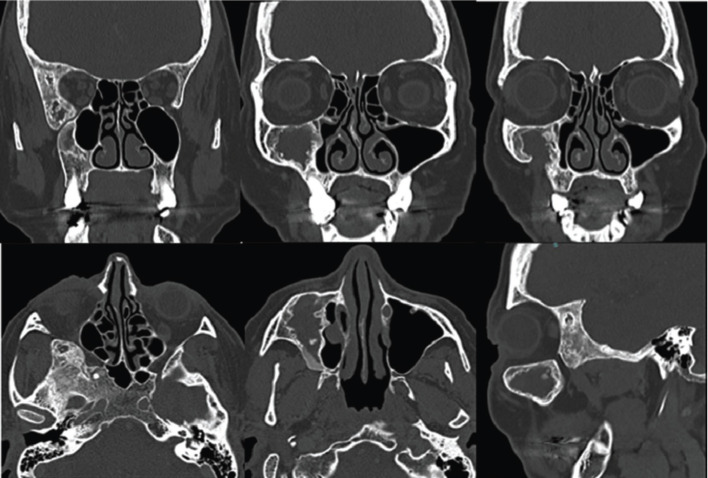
CT performed one year after the surgical procedure, the exam shows similar features.
